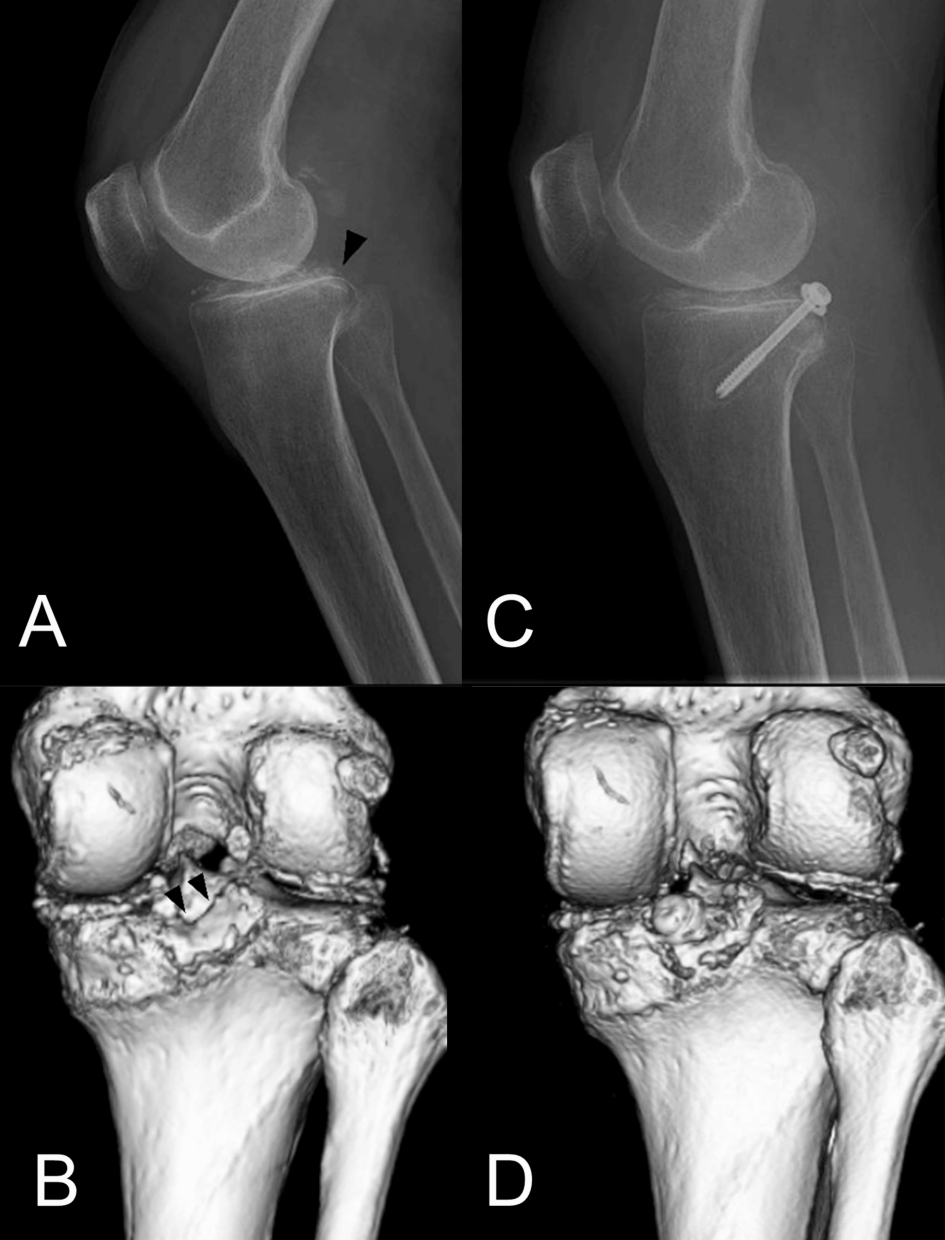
Case 2 (A) Preoperative lateral radiograph (arrowhead: bony fragment), (B) CT image (arrowhead: bony fragment). (C) Postoperative lateral radiograph and (D) CT image. CT, computed tomography; MRI, magnetic resonance imaging.
